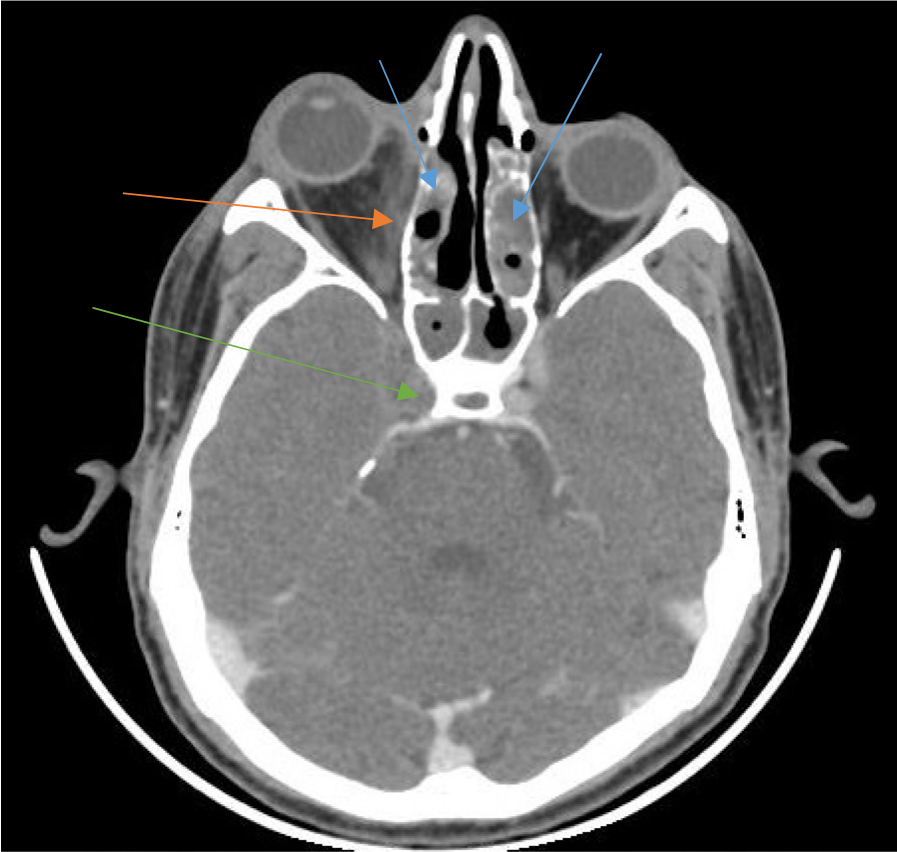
Axial section of contrast-enhanced CT of the brain in a patient with rhino-orbitocerebral mucormycosis showing non-enhancing mucosal thickening in bilateral ethmoid sinuses (blue arrows) with fat stranding in the medial aspect of the right orbit (red arrow). The cavernous segment of the right internal carotid artery is not opacified by the contrast as compared to the left side, suggestive of thrombosis (green arrow)
